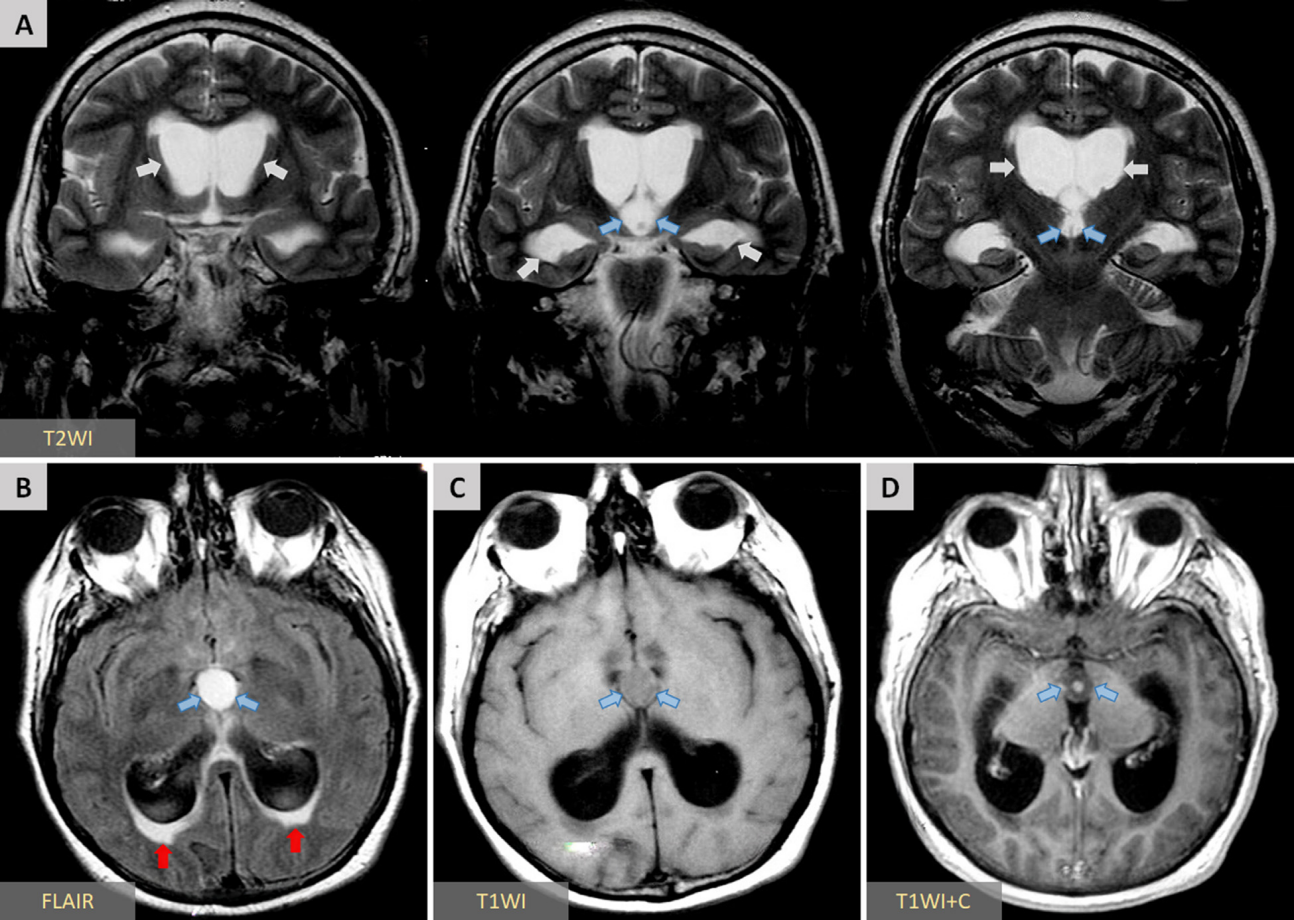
(A) Coronal T2-weighted MR images at different levels better characterize the described lesion as a well-defined fluid-filled cystic mass (*blue arrows*) located in the midline, in the topography of the anterior portion of the third ventricle, obstructing the foramina of Monro with resultant marked hydrocephalus (*white arrows*). (B) Axial FLAIR image demonstrates a still hyperintense signal exhibited by the described lesion (*blue arrows*), even with CSF signal suppression, postulating a higher protein content within the lesion compared to CSF. Some periventricular areas also exhibit hyperintense signals (*red arrows*), consistent with the transependymal flow caused by the existing obstructive hydrocephalus. (C) Axial T1-weighted MR image demonstrates an isointense signal exhibited by the lesion with no signs of enhancement postcontrast administration (*blue arrows*) (D).
